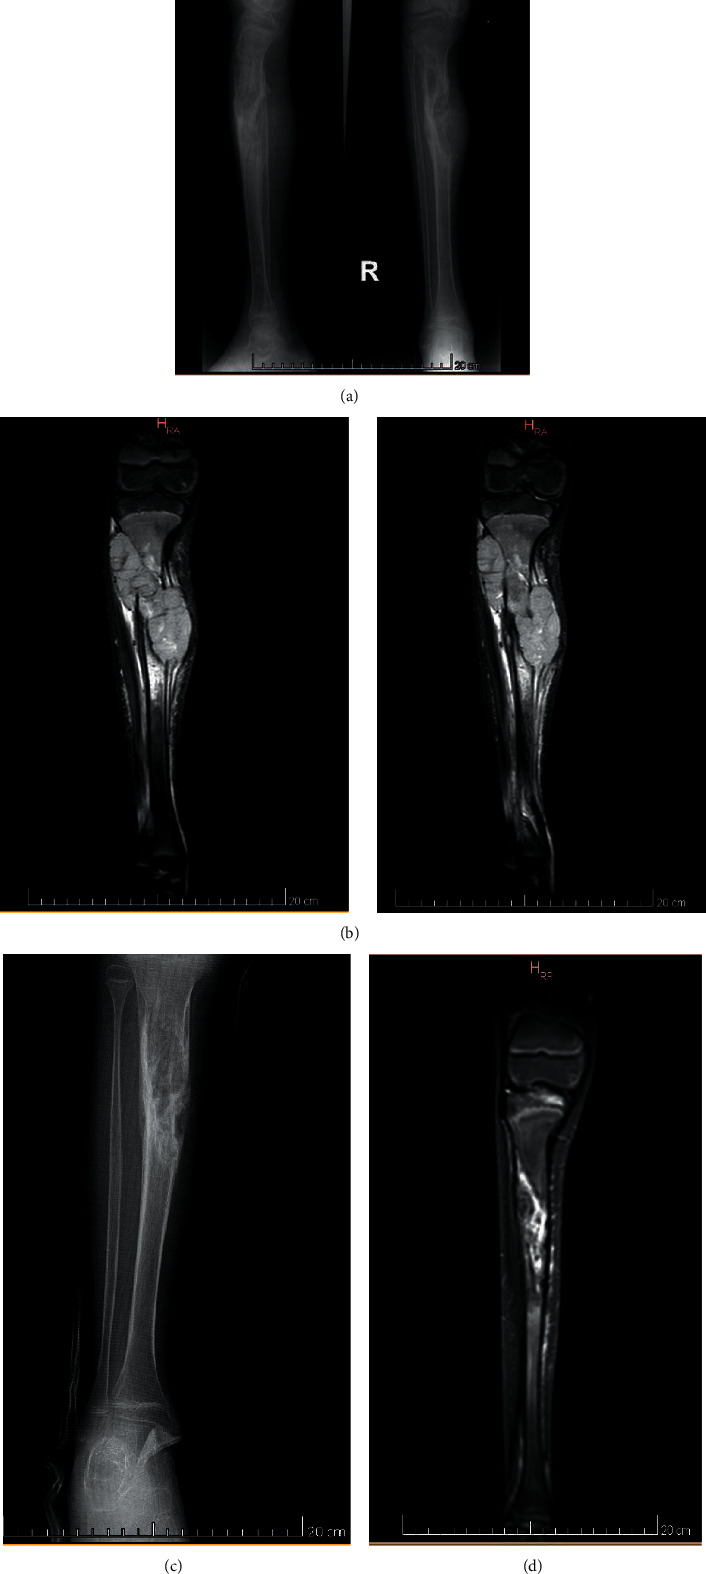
(a) X-ray at diagnosis, (b) MRI pretreatment, (c) X-ray posttreatment, and (d) MRI posttreatment.
